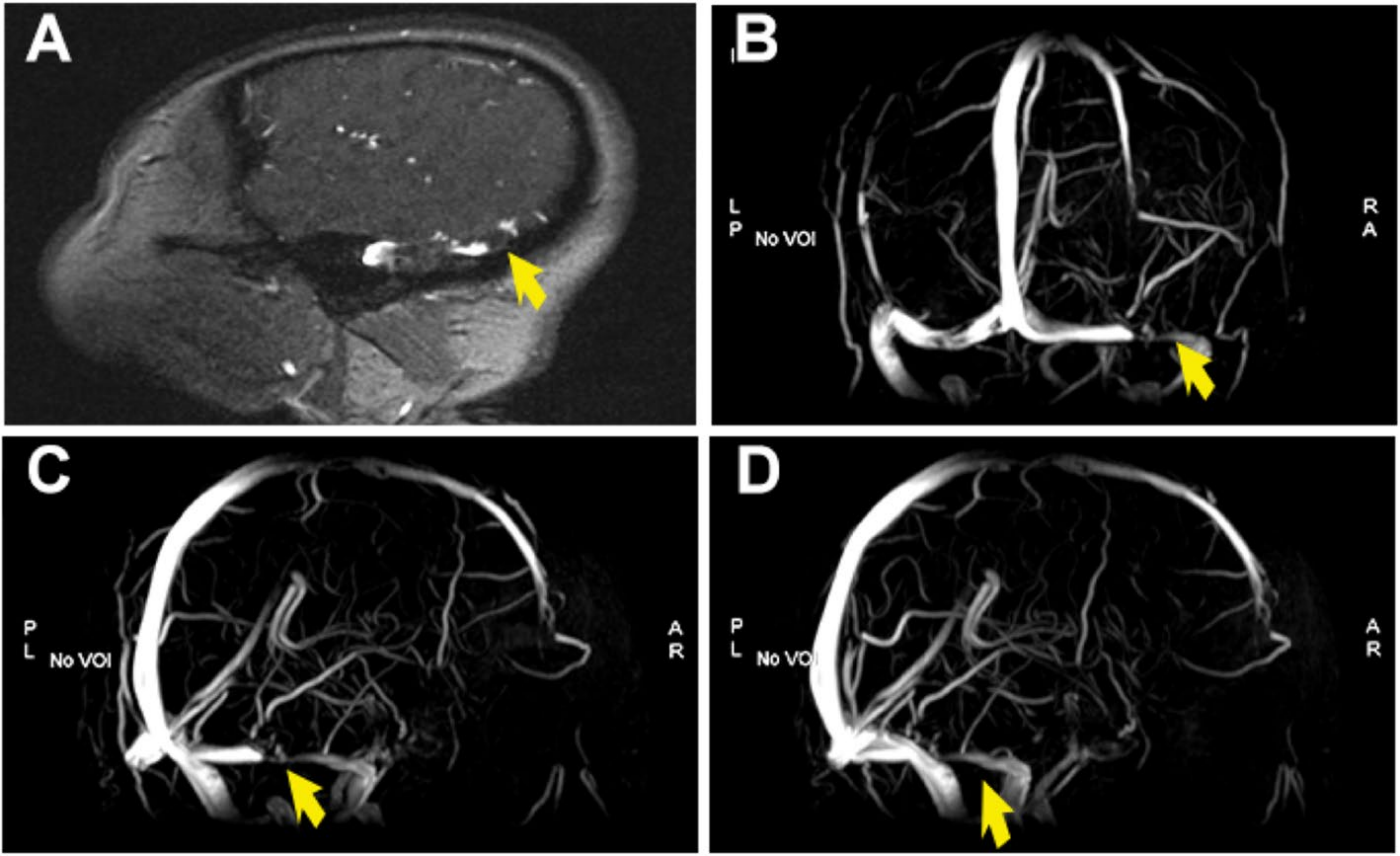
A-D Magnetic resonance imaging obtained at 1-month follow-up showed no significant change in the right transverse and sigmoid sinus (*arrow*)
